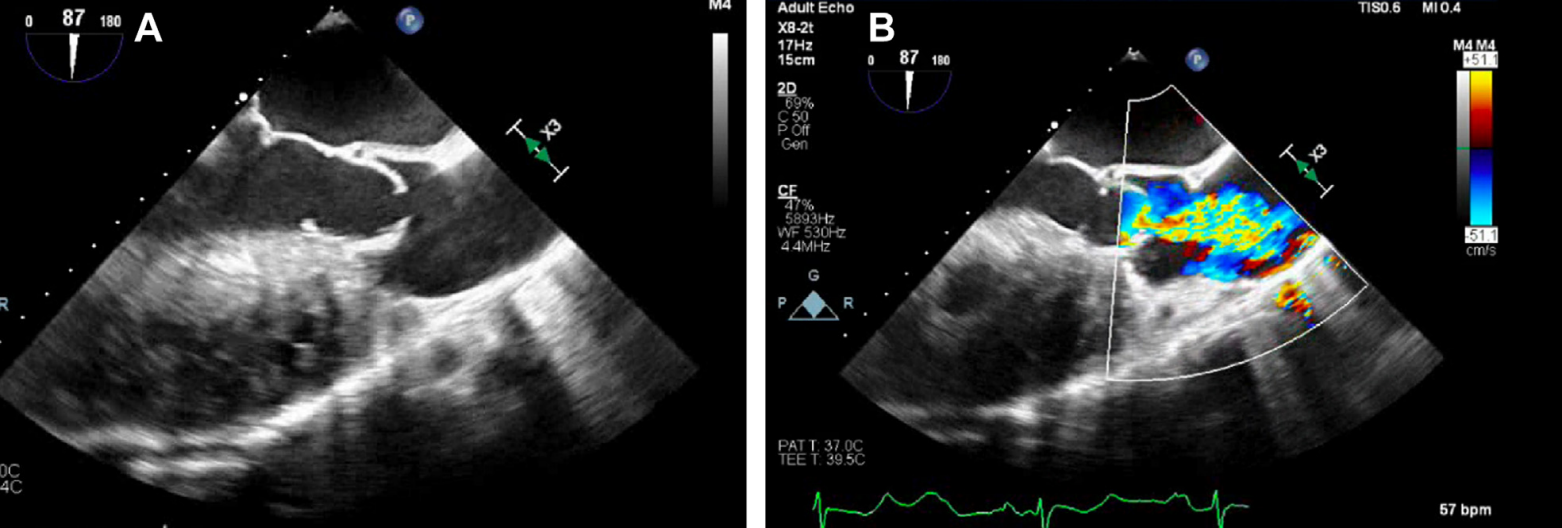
Transesophageal Echocardiography With Severe Pulmonary Stenosis This figure includes the transesophageal echocardiographic image depicting pulmonary valve doming with severe pulmonary stenosis in the patient before surgery. (A) 2-dimensional image; (B) color Doppler.
